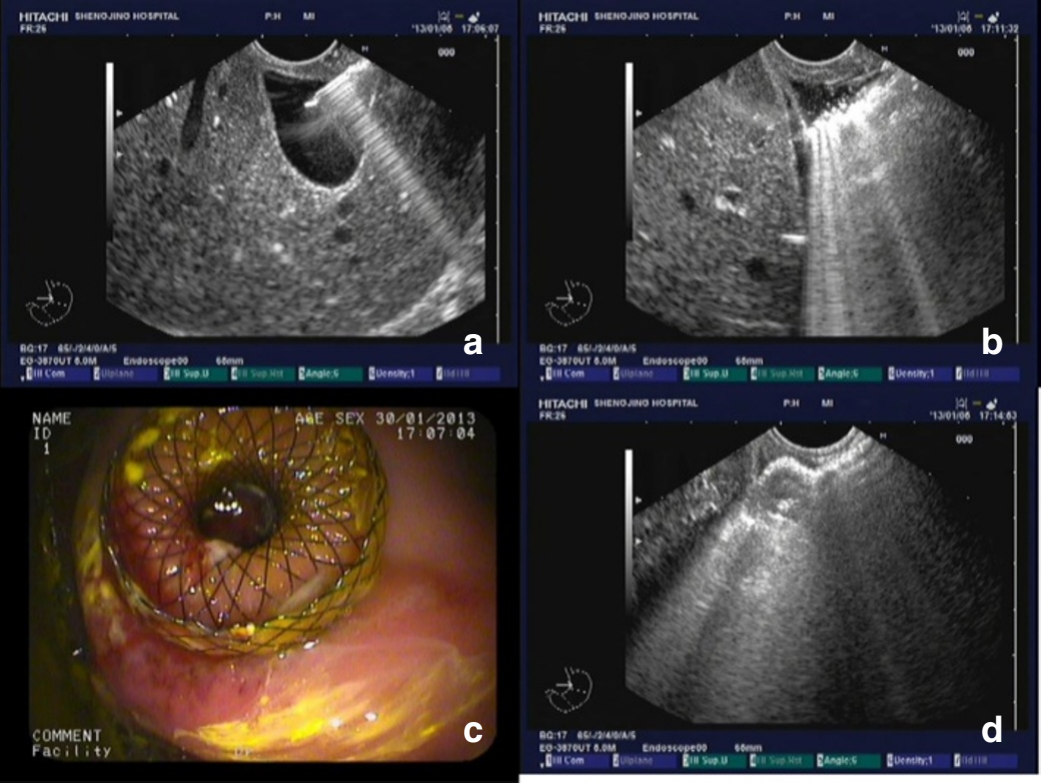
Cholecystogastrostomy. a. Endoscopy needle piercing the gallbladder. b. Dilation of the needle path using the cystotome, with a large amount of bile leakage. c. Endoscopic view of the deployed stent. d. Endoscopic ultrasound image of the deployed stent.
